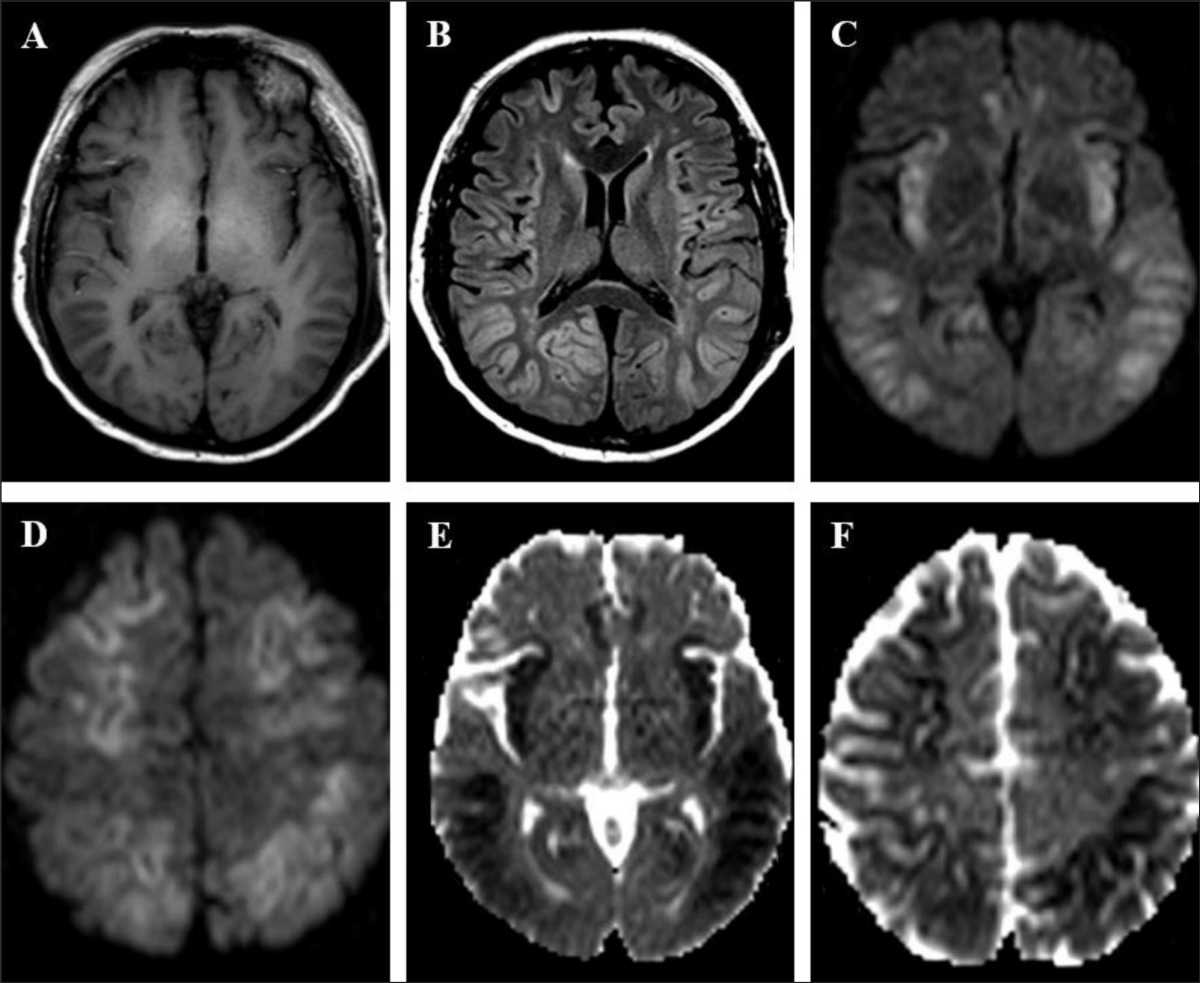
A 49-year old man was found in a coma. Clinical and laboratory findings indicated decompensated cirrhosis with encephalopathy. (A) T1-weighted imaging shows high signal in globi pallidi, suggesting underlying chronic hepatic disease. (B) FLAIR image shows bilateral and symmetric signal abnormalities in insular, cingulate, frontal and parietal cortices. (C, D) DWI (b-value = 1000 s/mm^2^) and (E, F) ADC show respectively high and low signal in affected cortices, consistent with cytotoxic edema, with sparing of occipital and peri-rolandic cortices.
